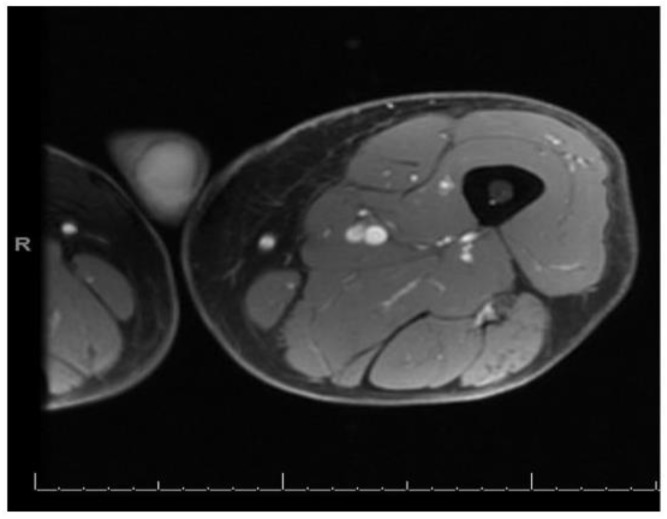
MRI of left thigh with resolution of diffuse edema and previous foci of multiple nodular and ring enhancing lesions.
